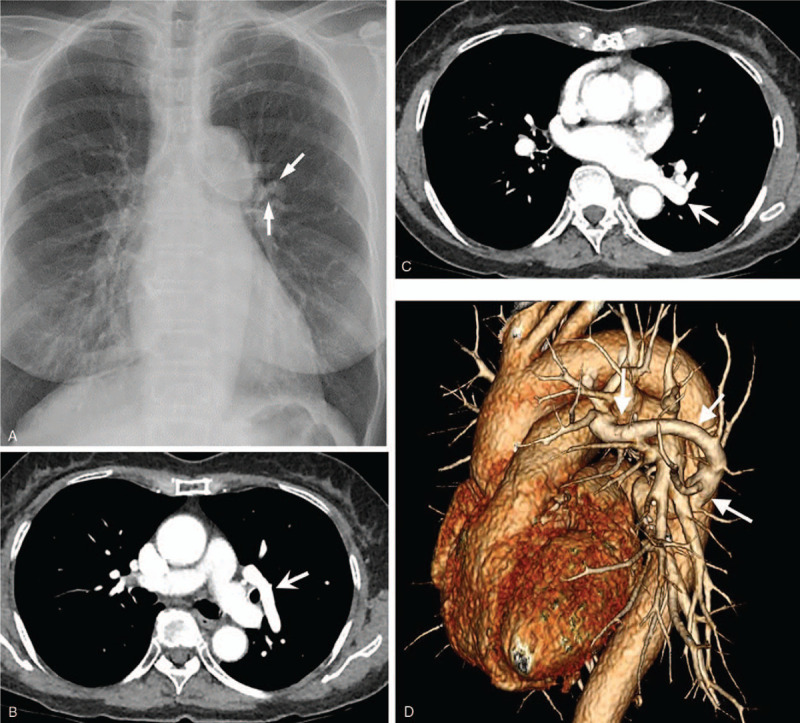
A 71-year-old woman presented with abnormal finding on chest radiograph during medical check-up. A. Chest radiograph showing a small dense curved opacity (arrows) in the left perihilar area. B and C. Chest CT images revealing an unusual vascular anomaly draining the left upper lobe (arrow in B) and joining the left inferior pulmonary vein (arrow in C) before merging into the left atrium. D. 3D volume rendered image showing an enlarged aberrant anomalous vein (arrows) courses tortuously to posterior direction and drains into the left atrium.
